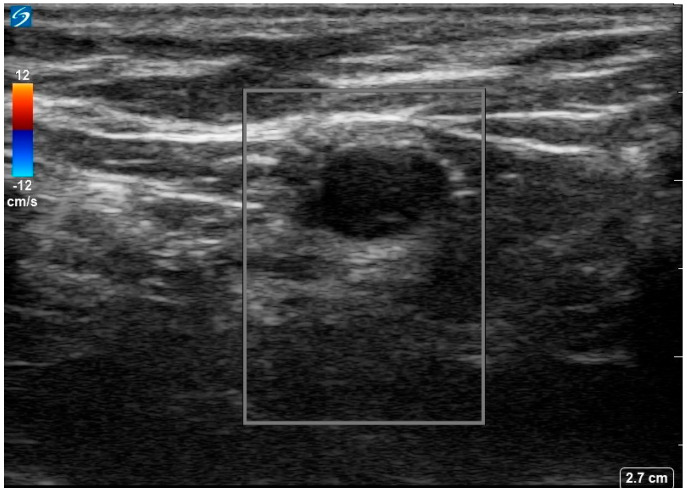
Ultrasound image of the 18−FDG avid left axillary lymph node. Image shows a 0.9 × 0.6 × 0.7 cm markedly hypoechoic lesion with loss of normal fatty hilum, reniform shape, and irregular margins with prominent peripheral feeding vessels.
